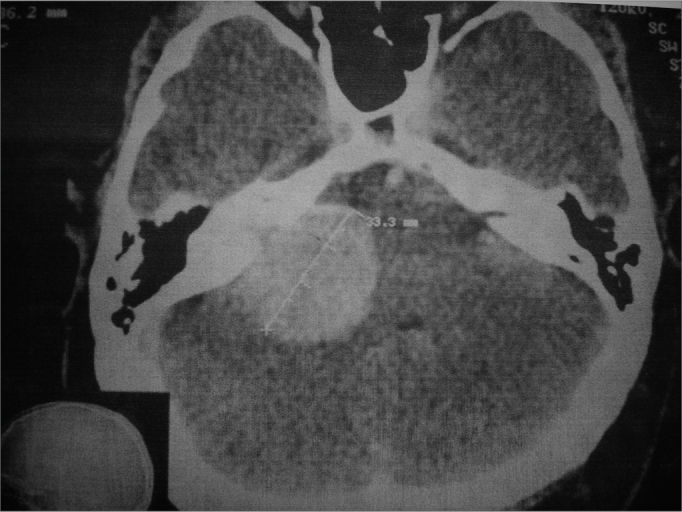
Mastoid CT scan showing a globus lesion (33.3mm in its largest axis), extending to the internal auditory meatus and enlarging it. We also noticed mass effect and compression of the cerebellum and pons.
